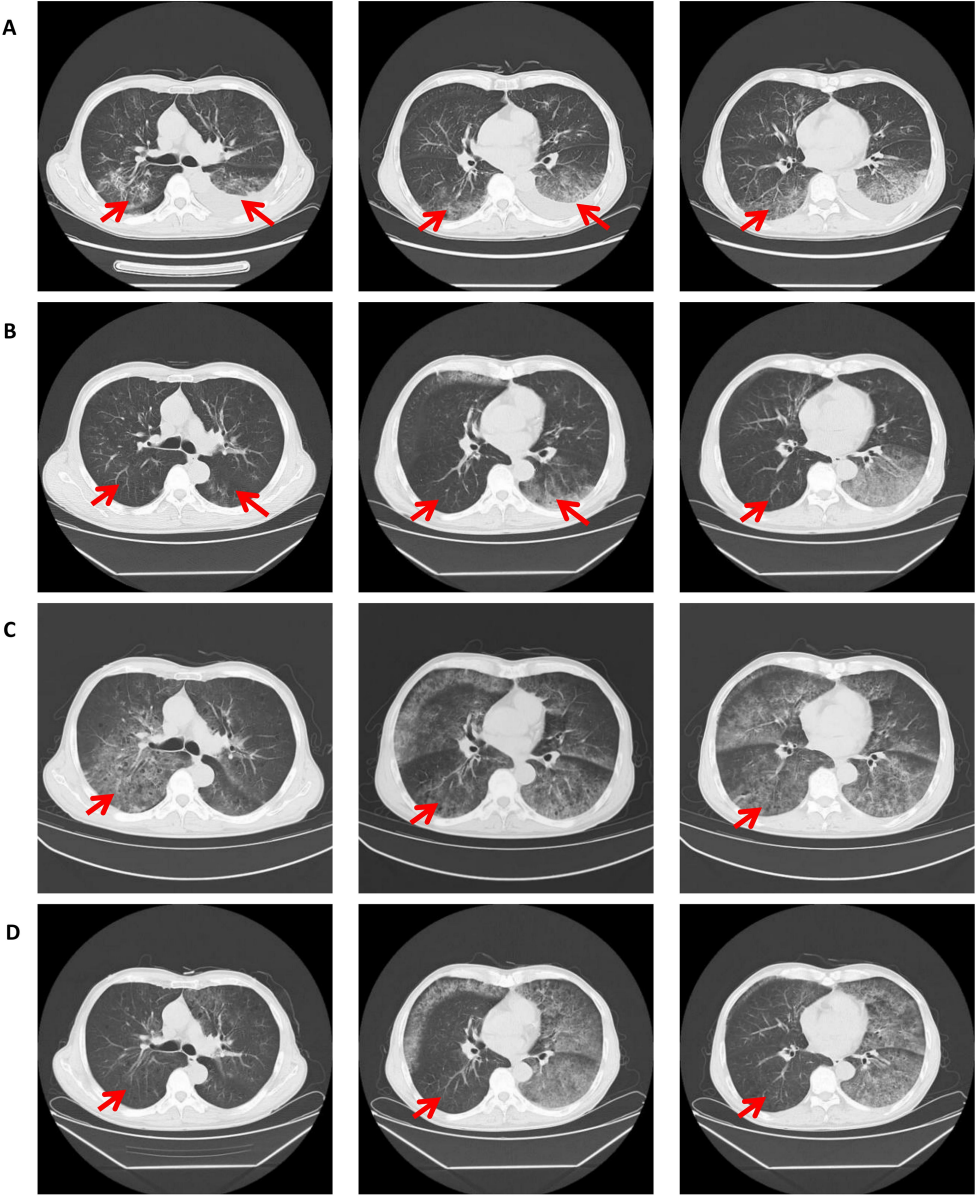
Serial computed tomography (CT) imaging of the chest demonstrates the dynamic radiographic changes in response to sequential therapies. (A) Scan after 5 days of furmonertinib therapy shows early radiographic improvement. (B) Following 45 days of continuous furmonertinib, a significant radiographic improvement is evident. (C) Subsequent imaging after 2 cycles of platinum-based chemotherapy combined with sintilimab demonstrated disease progression. (D) Re-challenge with furmonertinib for 5 days shows early radiographic improvement.
